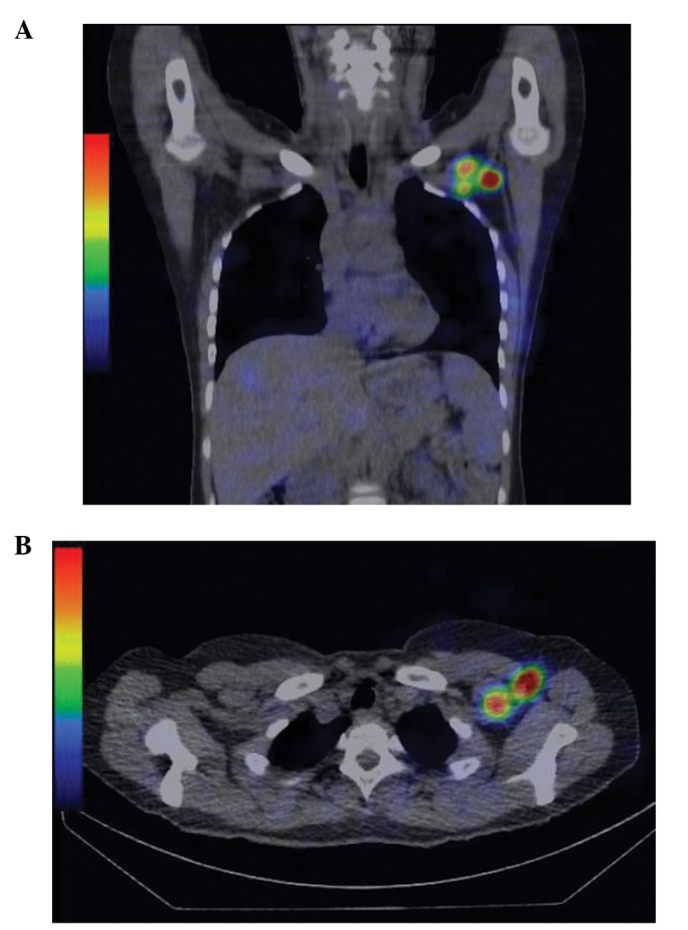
SPECT/CT scans of a patient in the left breast. Each shows the CT scan in the (A) coronal and (B) transaxial. In the fused images, the axillary SN is in color at levels I and II. SPECT/CT, single-photon emission computed tomography/computed tomography; SN, sentinel nodes.
